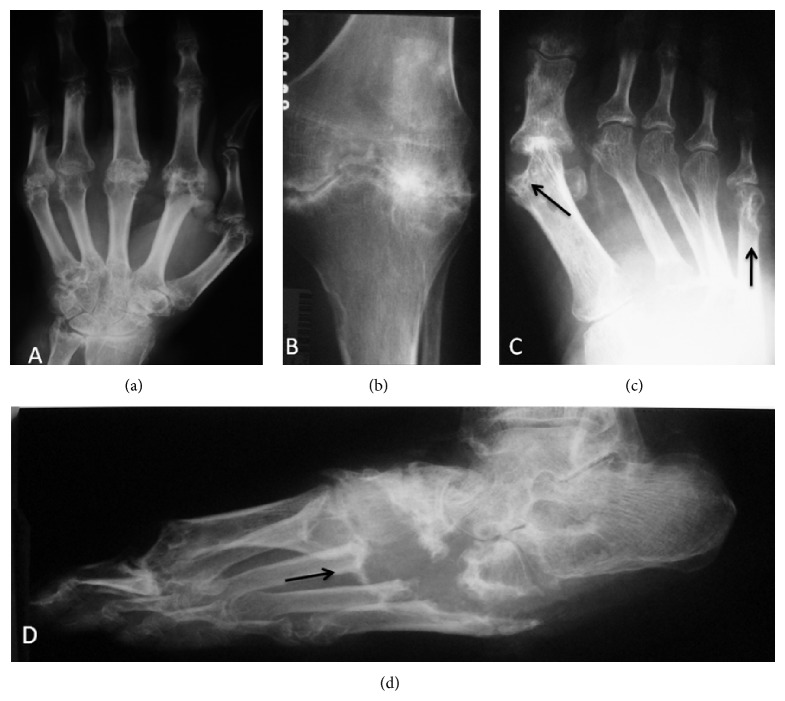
Radiographs of hand (a), knee (b), foot (c), and ankle (d) showing severe destructive changes secondary to tophaceous gout with erosion, soft tissue swelling (tophi), articular space narrowing, and proliferative bone abnormalities. Note the well-defined extra-articular large erosions with overhanging margin and surrounding bone eburnation (arrows). The uniform joint space narrowing with normal bone density in knee and wrist is typical of late gout disease.
